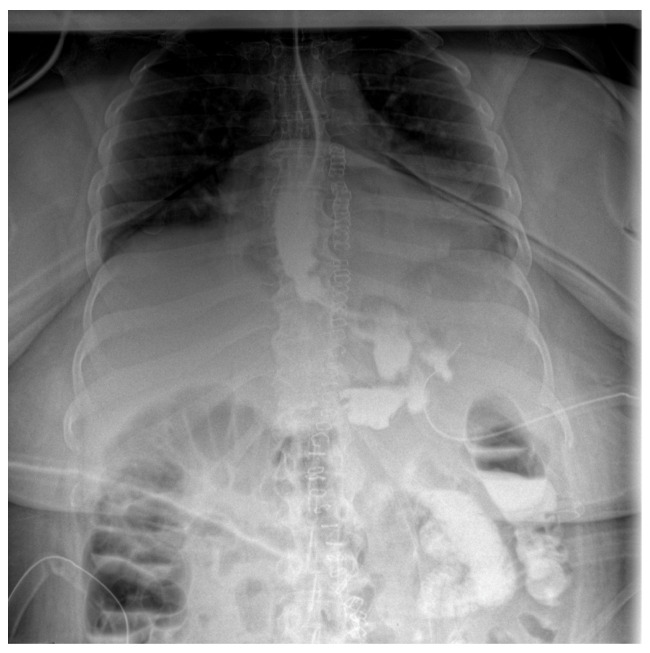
Contrast abdominal radiography—with E-VAC in place without leakage of the contrast substance.
